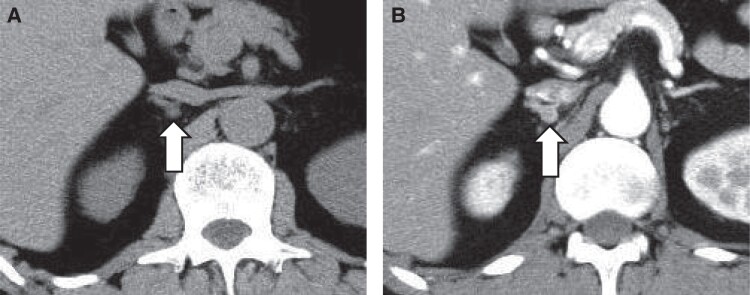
(A) Plain CT image and (B) iodine contrast CT image showing a right adrenal nodule (8 × 7 mm) with CT attenuation values of < 10 HU. Abbreviation: CT, computed tomography.
